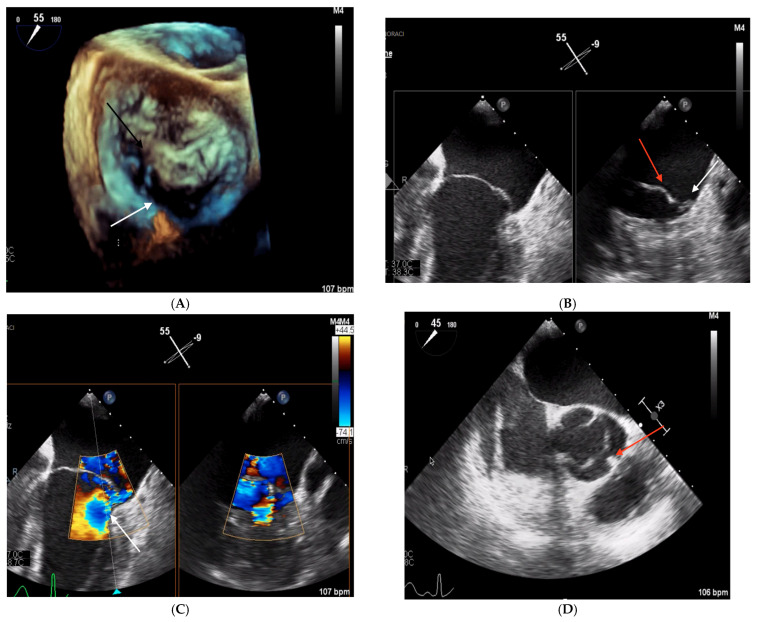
TEE images obtained in Clinical Case 1. (A) 3D TEE “en face” view of mitral valve showing a severely hypoplastic PML (white arrow) and an elongated and prolapsing AML (black arrow). (B) 2D TEE image at 55° mid-esophageal level (left panel) showing the elongated AML. The X plan in A1P1 (right panel) shows a large AML (red arrow) and severe PML hypoplasia (white arrow). (C) Color Doppler 2D TEE 55° mid-esophageal view (left panel) showing the severe, eccentric MR jet (white arrow) and the orthogonal plane crossing A1/P1, where the origin of the MR jet is located (right panel). (D) 2D TEE image at 45° showing type 1 L-R bicuspid aortic valve. The red arrow indicates cup fusion.
